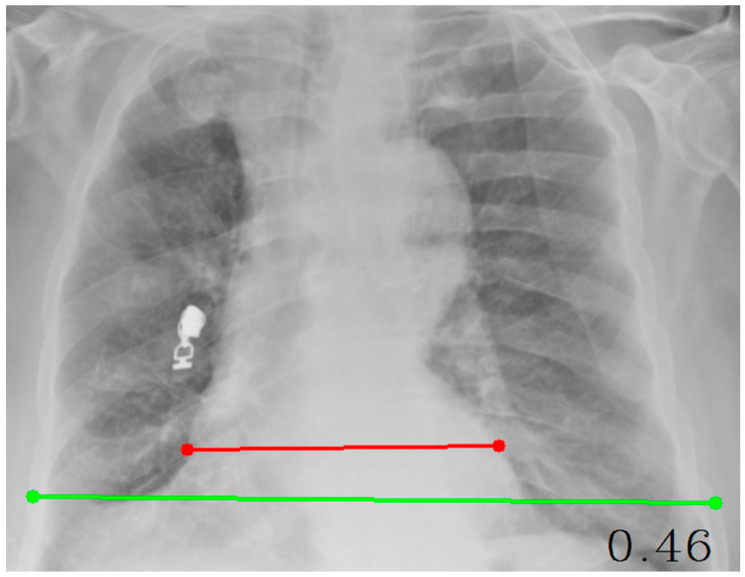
Measurement of the Cardiothoracic Ratio (CTR) on a Posteroanterior Chest Radiograph.
